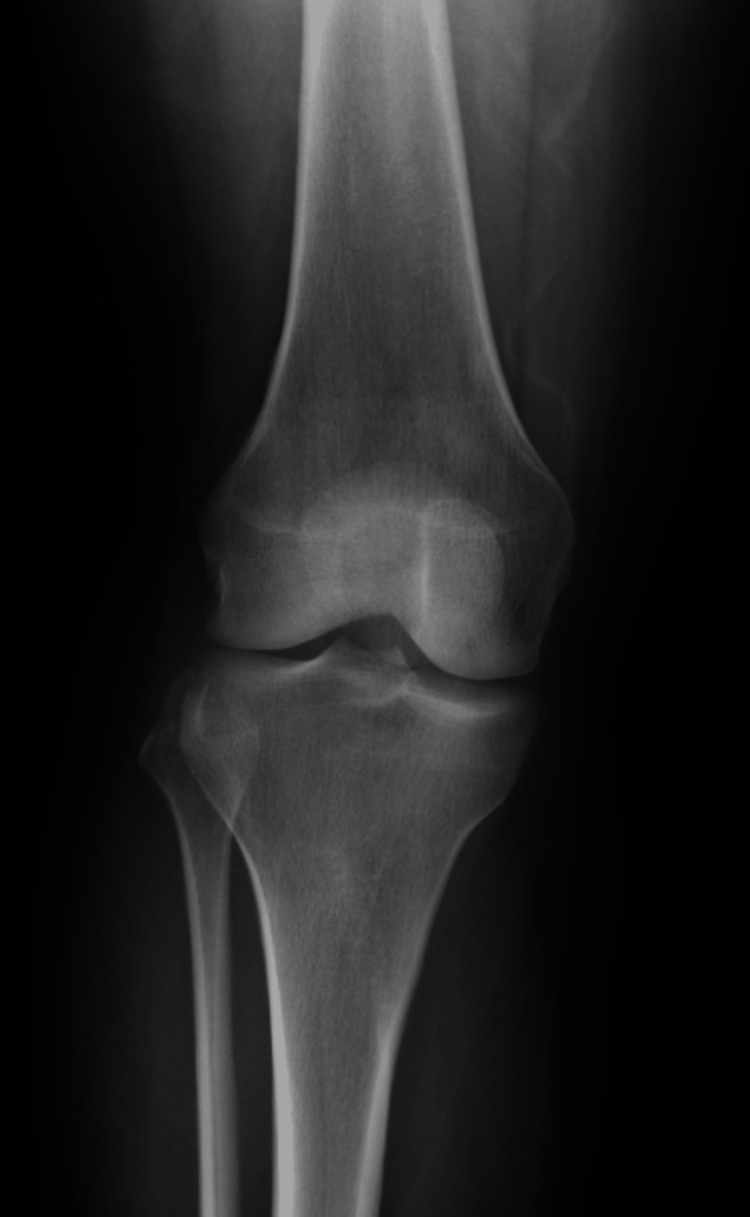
AP radiograph of the patient’s right knee a few hours after injury AP: Anteroposterior
